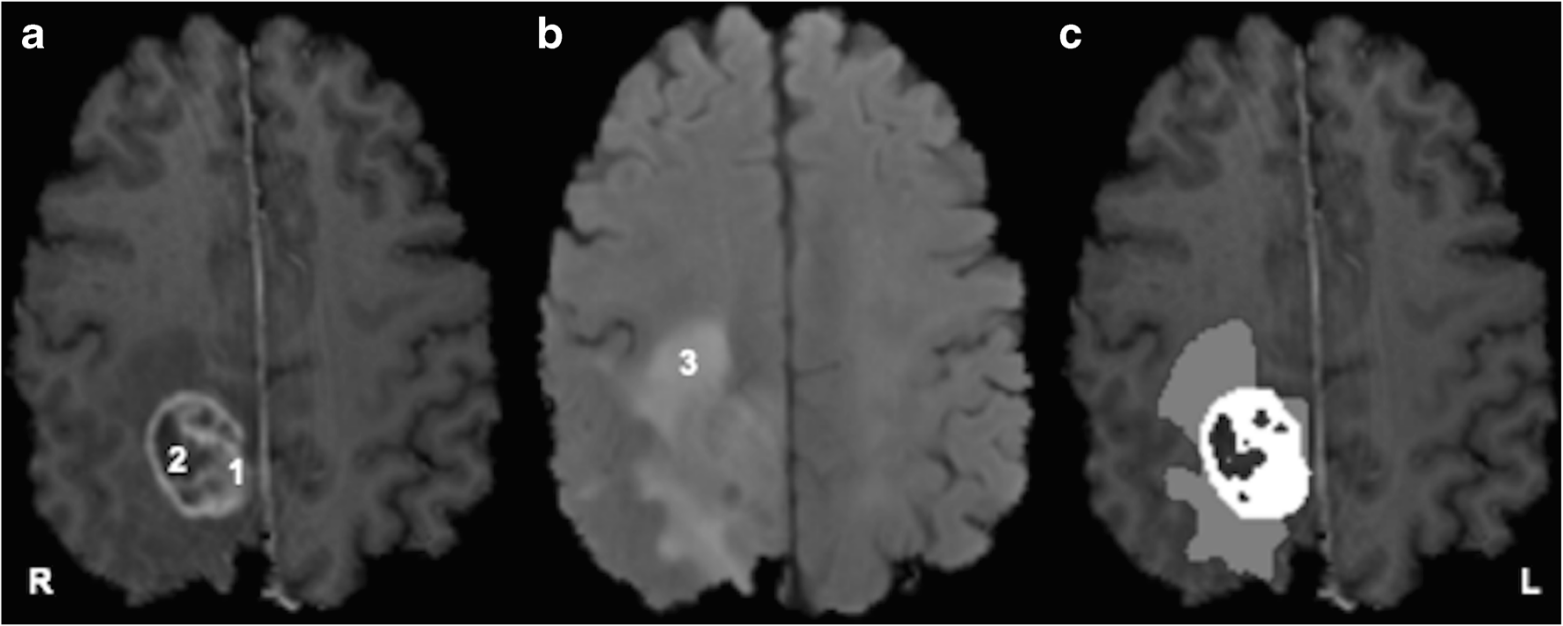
Example segmentation showing tumour subregions and imaging sequences. a T1-post-contrast. b T2 fluid-attenuated inversion recovery (FLAIR). c Whole-tumour mask. 1 = contrast-enhancing region = blue; 2 = necrotic core; 3 = peritumoural oedema. R = right. L = left
